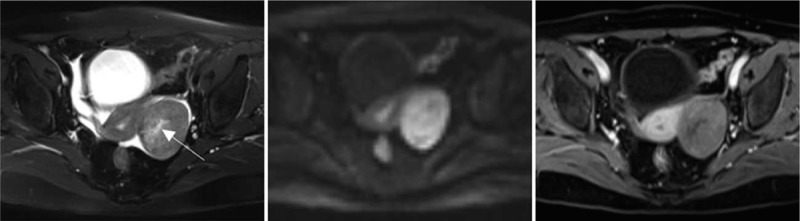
Left ovarian thecoma in a 68-year-old woman. (2-1) Cross-sectional T_2_WI showed that the lesion is located in the left posterior uterus. The edge is clear, elliptical, and a likely flocculent cyst change (arrowhead). A small amount of free effusion can be seen in the pelvic cavity. (2-2) B value of the DWI sequence of 1000 s/mm ^2^ showed a hyperintense signal with diffuse restriction and clear edges. (2-3) Enhanced scanning, with lesions showing uneven and mild enhancement; no enhancement was seen in the cystic zone.
